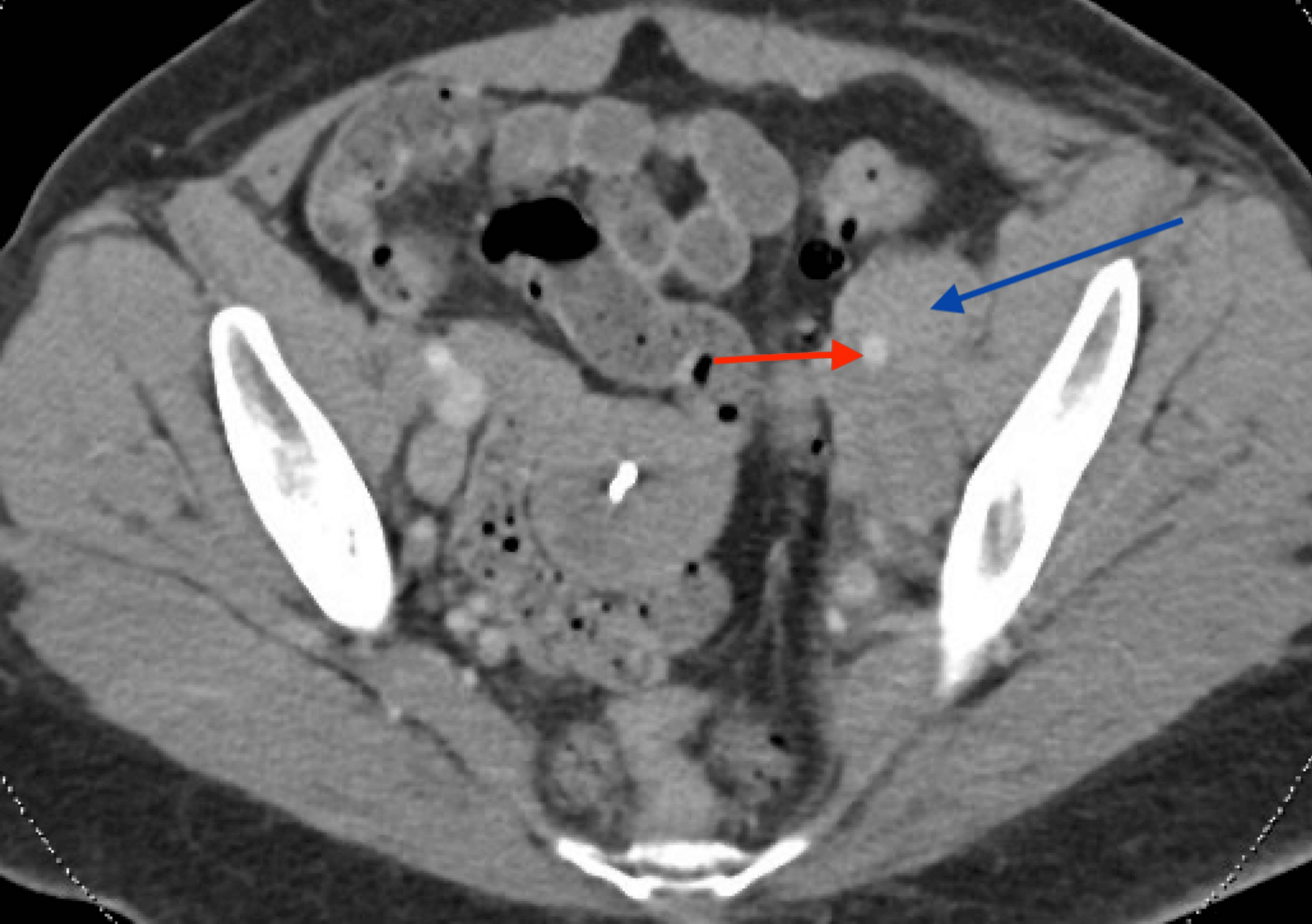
CT scan of the abdomen and pelvis Red arrow shows external iliac artery. Blue arrow shows confluent lymph nodes measuring 61x35 mm.
